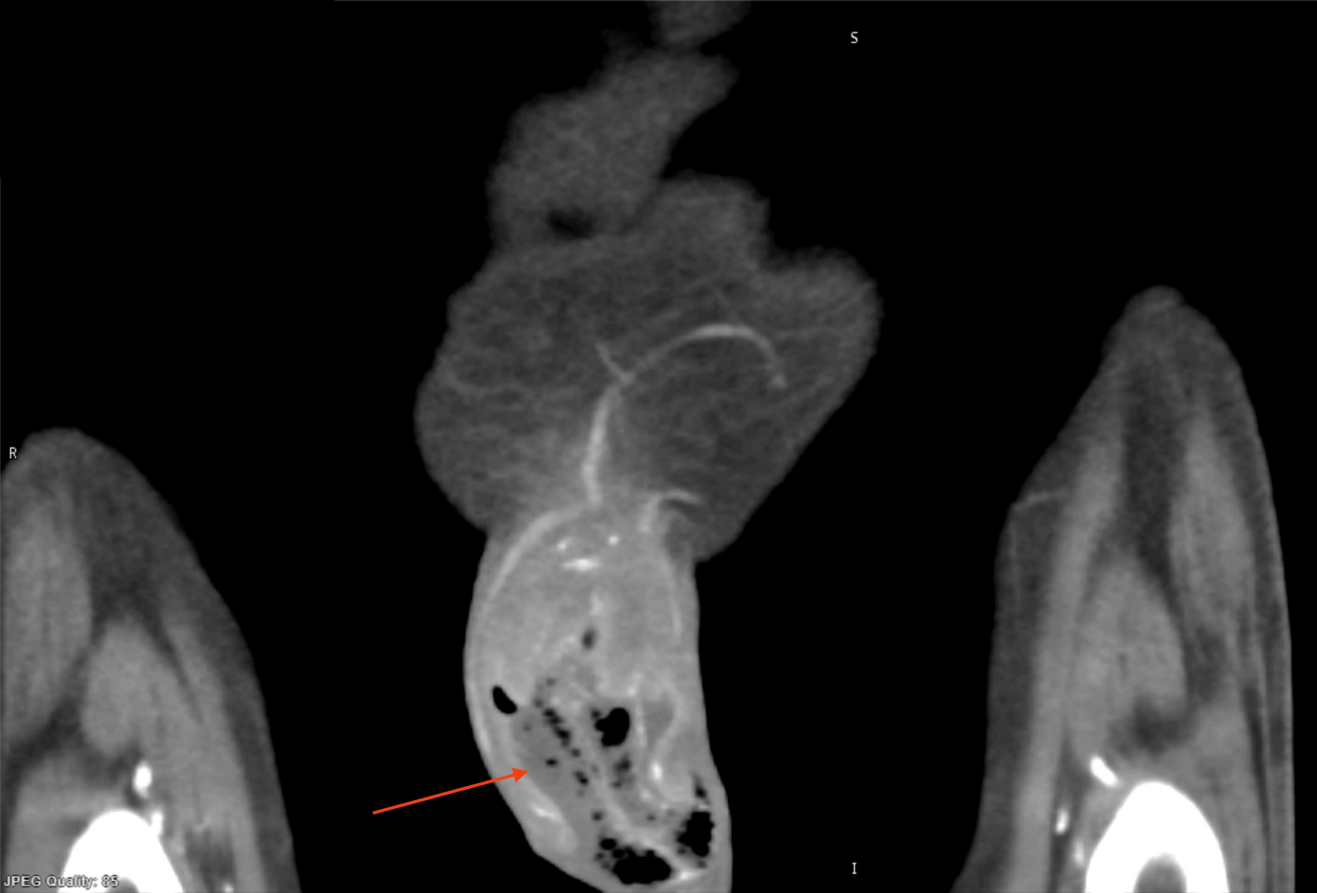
Coronal CT image of the abdomen and pelvis demonstrating a fluid and air collection (arrow) within the distal corpus cavernosum of the penis, with associated penile edema, findings consistent with extensive soft tissue necrosis and abscess formation CT: computed tomography
